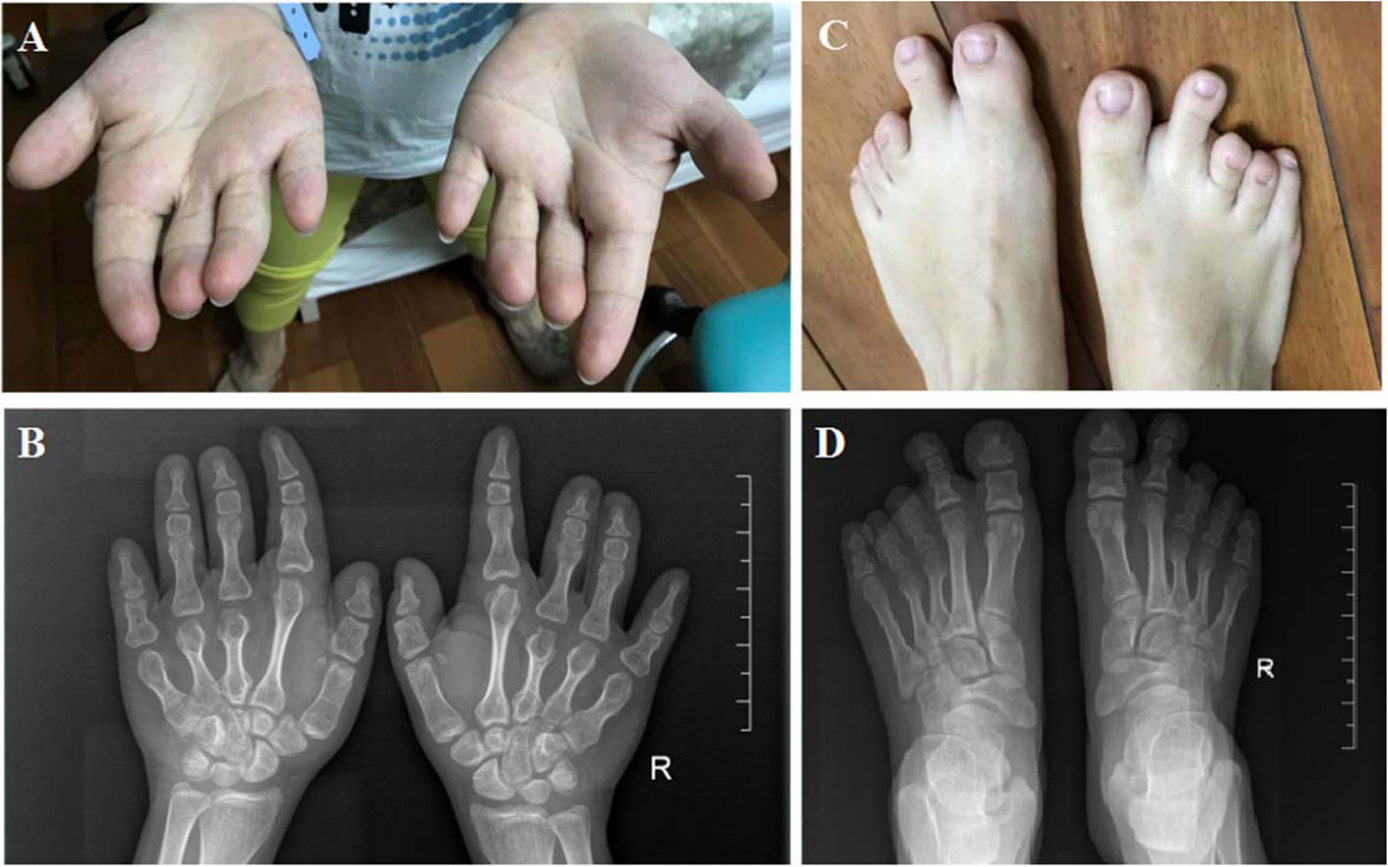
a–d The appearance and radiological findings of the proband with brachydactyly type A1(BDA1). a showing shortened fingers and absence of middle phalanges of the fifth finger and radial deviation of the second finger. b Radiographic images of proband’s hand: shortening of the middle phalanges of digits II–V, fusion of middle and terminal phalanges of 5th finger, bilateral shortening of metacarpals bone 3–5. c showing short toes and absence of middle phalanges of the third to fifth toes. d Radiographic images of proband’s foot: fusion of middle and terminal phalanges of third to fifth toe, bilateral shortening of metatarsals bone 3–4
